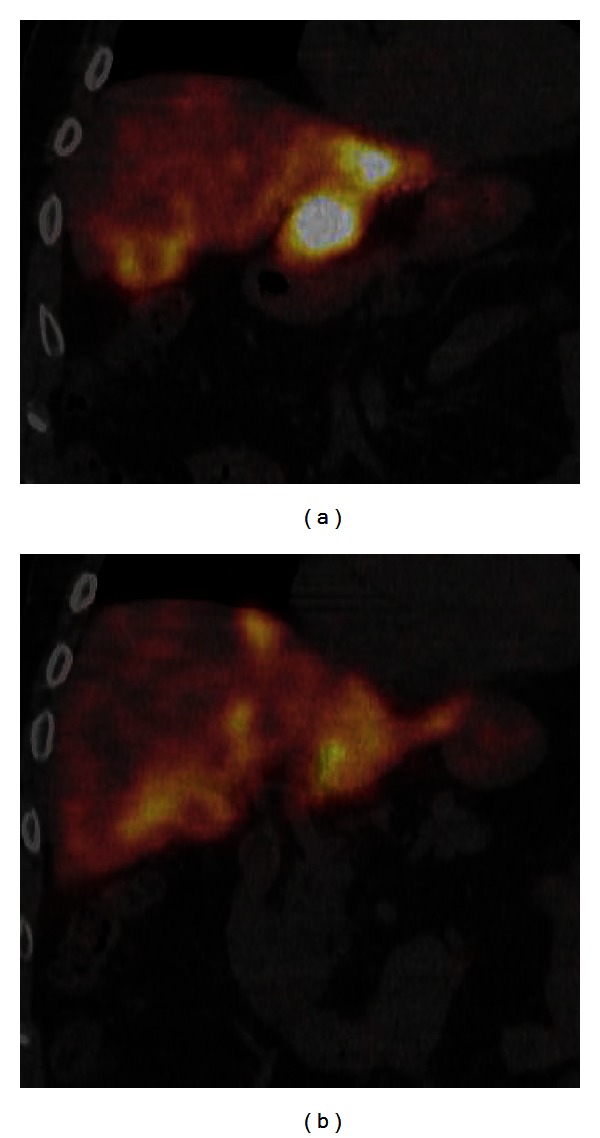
(a) and (b) Coronal fused SPECT/CT MAA study demonstrates multifocal hepatic uptake without gastrointestinal activity.
